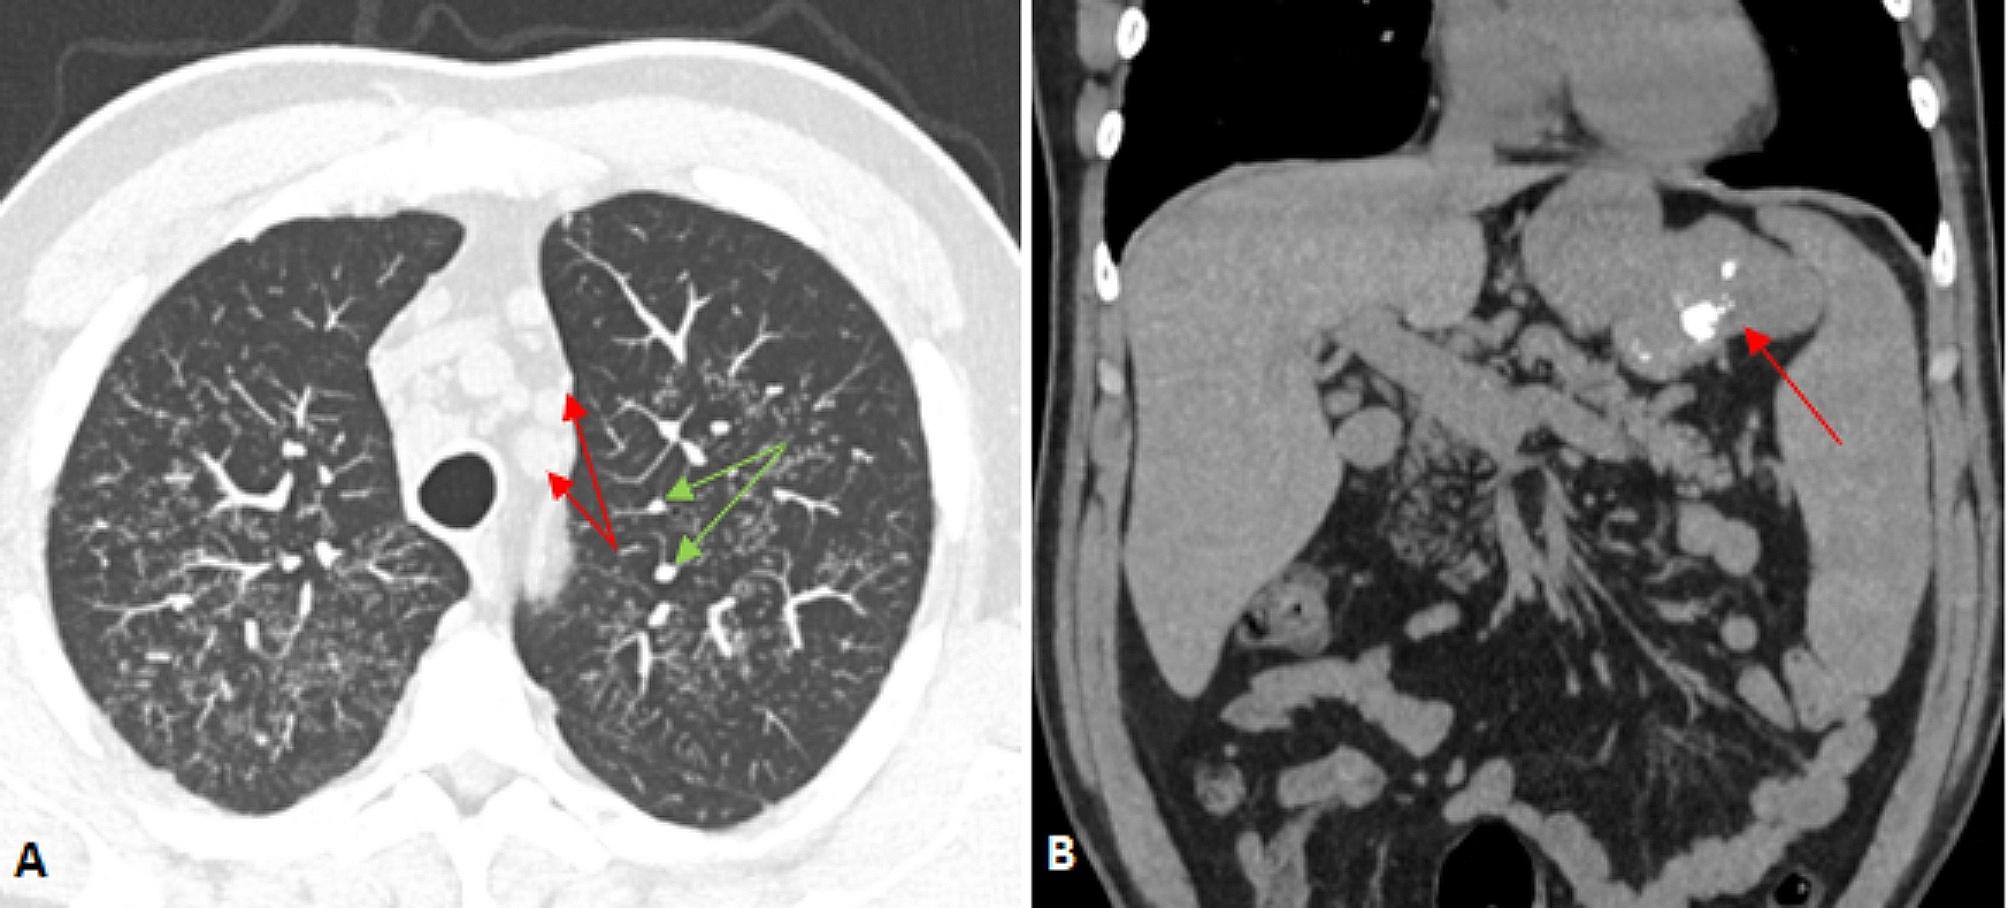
(A) Computed tomography chest demonstrating mediastinal lymphadenopathy (red arrows) and peri-lymphatic micronodules (green arrows) (B) Computed tomography abdomen demonstrating coalescent mass between stomach and spleen with calcification (red arrow)
